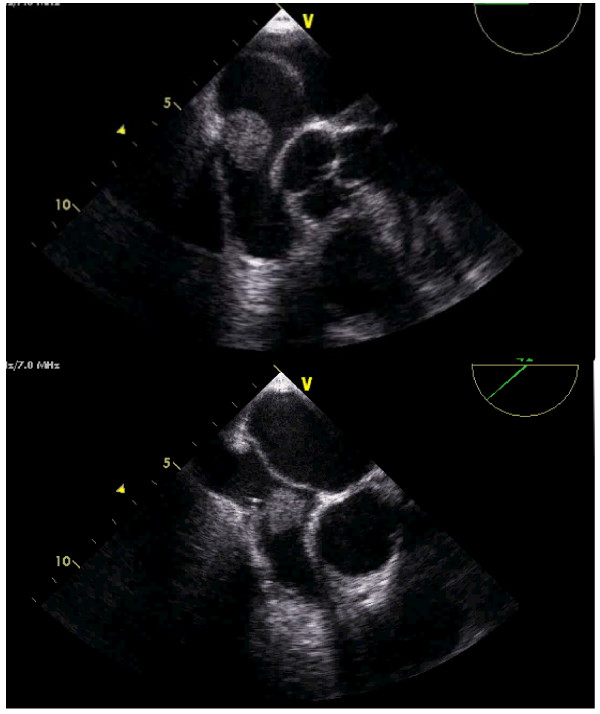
Transesophageal echocardiography. Huge right atrial mass. Note the hypermobile interatrial septum and the tip of the catheter in its proximity.
